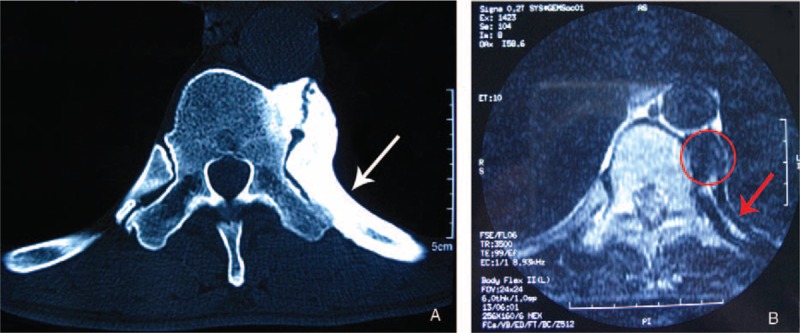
(A) Noncontrast axial chest computerized tomography (CT) scan showing irregular sclerosis of the diaphysis of the 10th rib (white arrow), with periosteal reaction and narrowing of the medullary cavity. (B) Axial T1-weighted MRI at the level of 10th thoracic vertebra showing heterogeneous low signal intensity over the 10th rib (red arrow). Sclerosing osteophytes can be seen on the left side of the 10th thoracic vertebra (circle).
